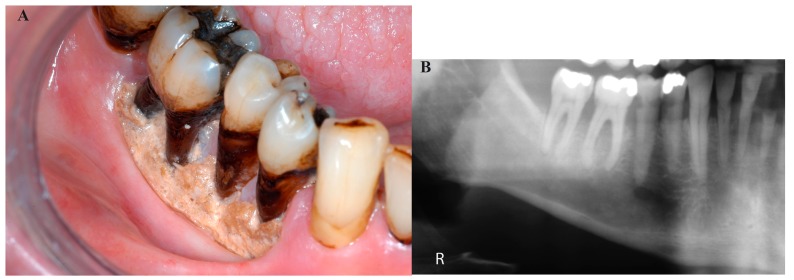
Stage I; exposure of mandibular bone (A); no distinct radiographic changes on the panoramic view (B).
